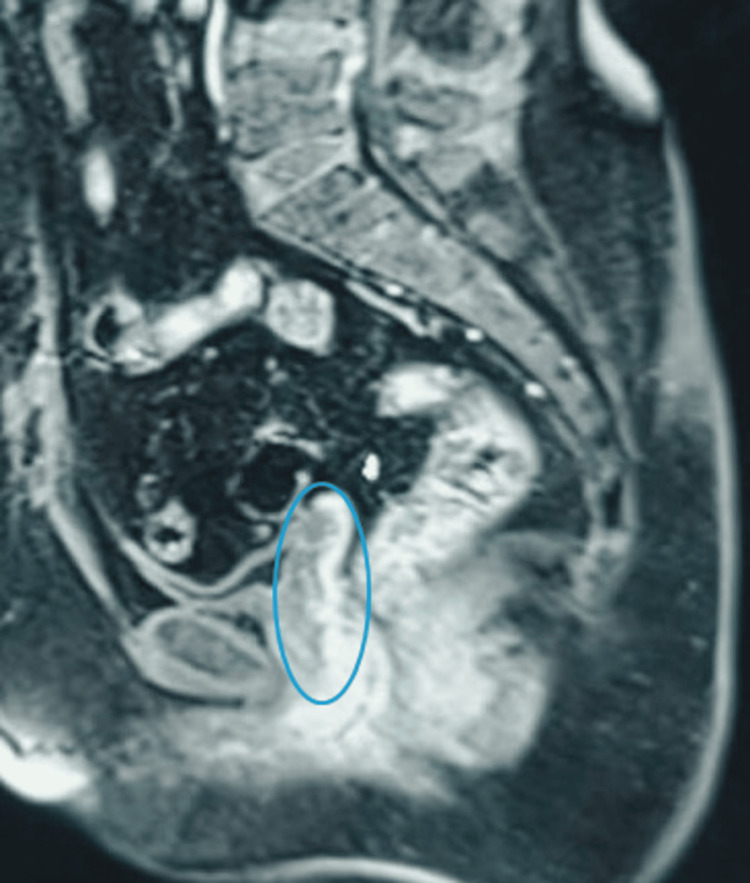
Sagittal T2-weighted MRI of the pelvis showing anterior vaginal wall mass Sagittal T2-weighted MRI of the pelvis showing a well-defined polypoidal mass arising from the anterior vaginal wall (encircled) without adjacent organ invasion.
